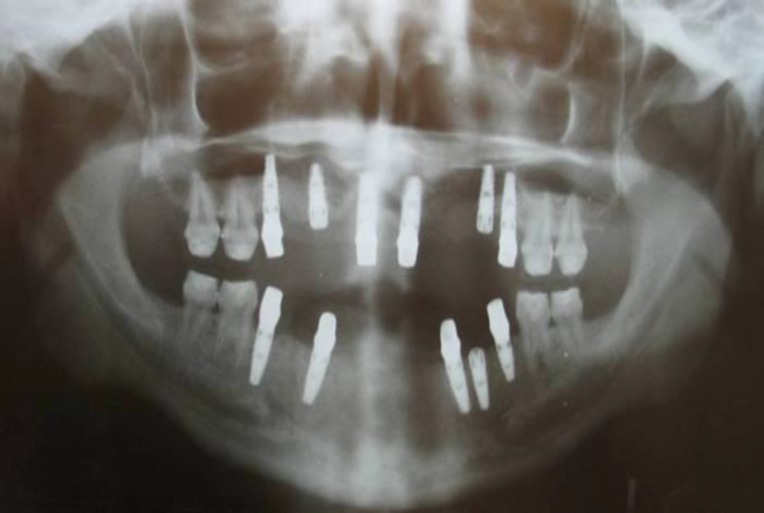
Postoperative panoramic radiograph of osseointegrated implants
